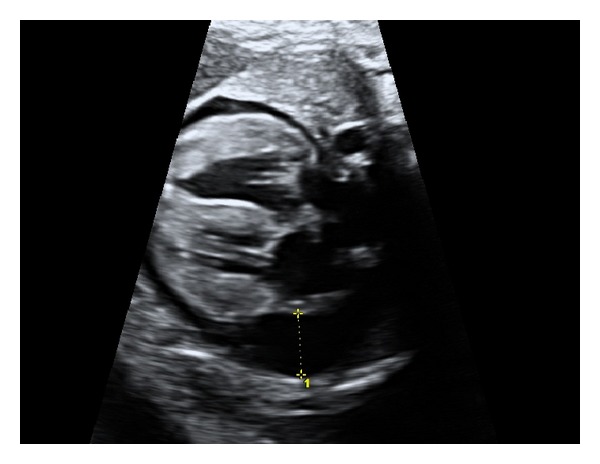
Fetal echocardiography at 37 weeks shows cardiac hypertrophic involving the right ventricular, left ventricular and interventricular septum, and pericardial effusion.
